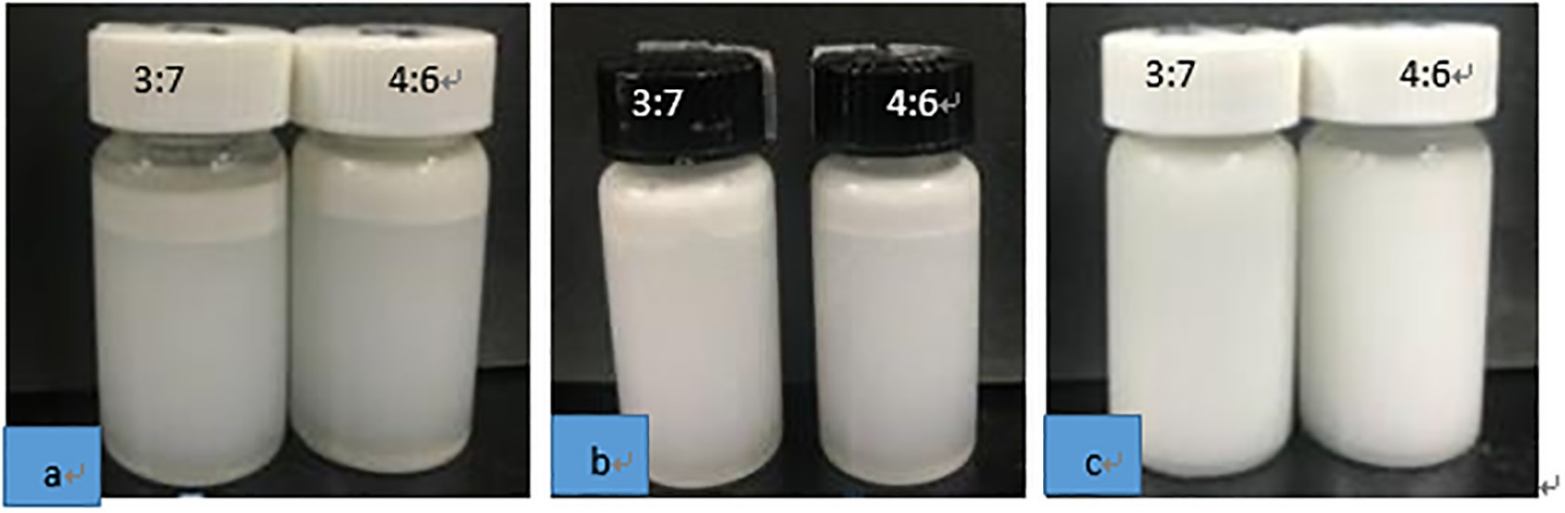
Photographs of 5% coconut o/w emulsions made by different methods. Emulsions were stored at room temperature for 30 days. a, high-pressure homogenization treatment, 30MPa; b, high-pressure homogenization treatment, 50MPa; c, US treatment, ultrasonic power of 270 W.
